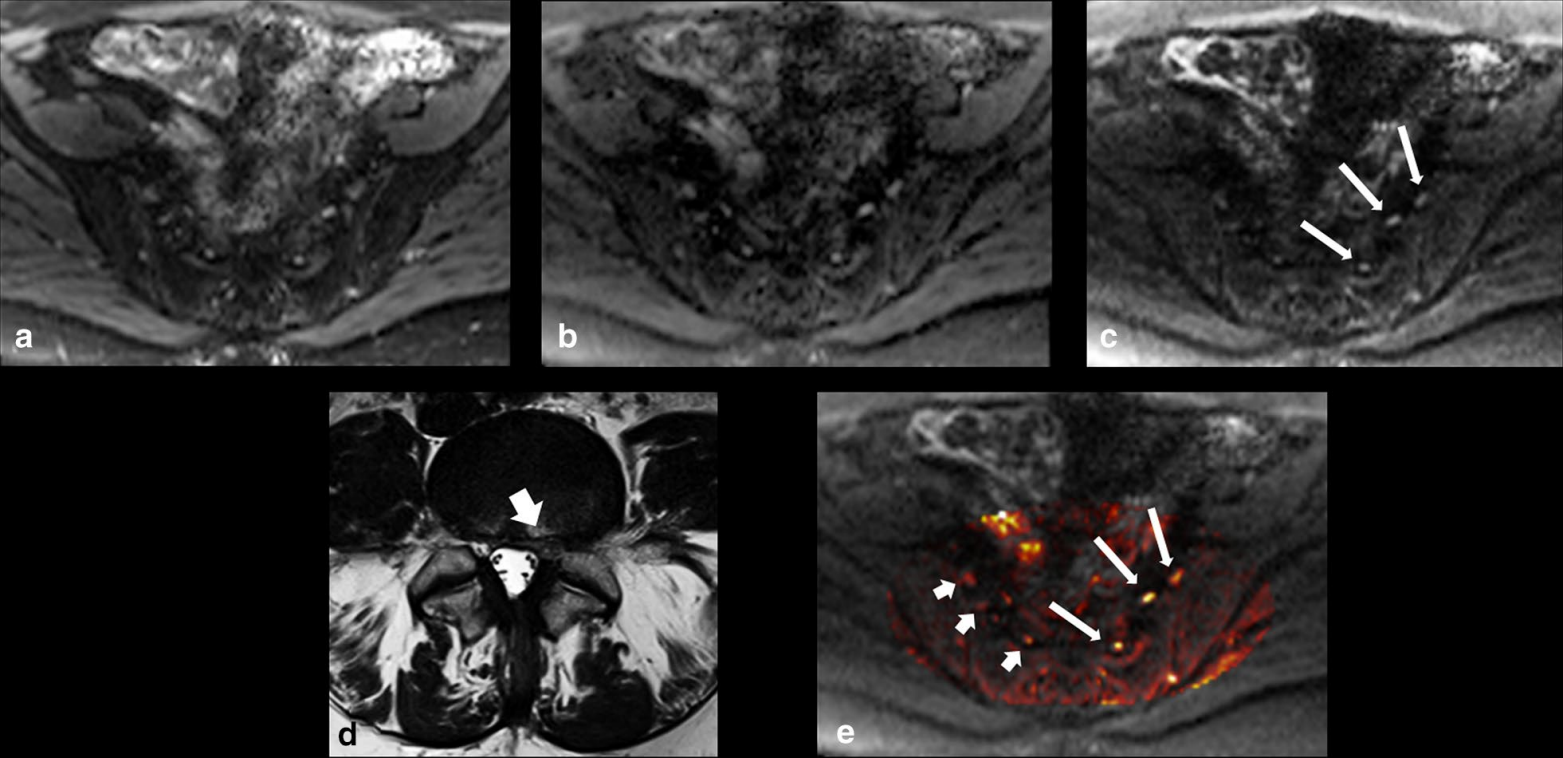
38-year-old female who presented with left leg pain and numbness. DWI-RESOLVE images (a) *b* = 50, (b) *b* = 500, (c) *b* = 800. The arrows on the b800 image correlate to the left L5, S1 and S2 nerves from anterior to posterior. The nerves were evaluated by both readers on axial DWI-RESOLVE images as positive for abnormality (grade II abnormal size and signal intensity). The left L5 nerve appears less involved compared to the left S1 and S2 nerve levels. Axial T2W TSE (d) of the lumbar spine at L5/S1 highlights a large left-sided disc herniation (arrow), which explains symptoms and correlates to nerve abnormalities. The color-mapped image e demonstrates the asymmetry of the lumbosacral plexus to better effect. Long arrows indicate abnormal left nerves and short arrows indicate normal contralateral side
